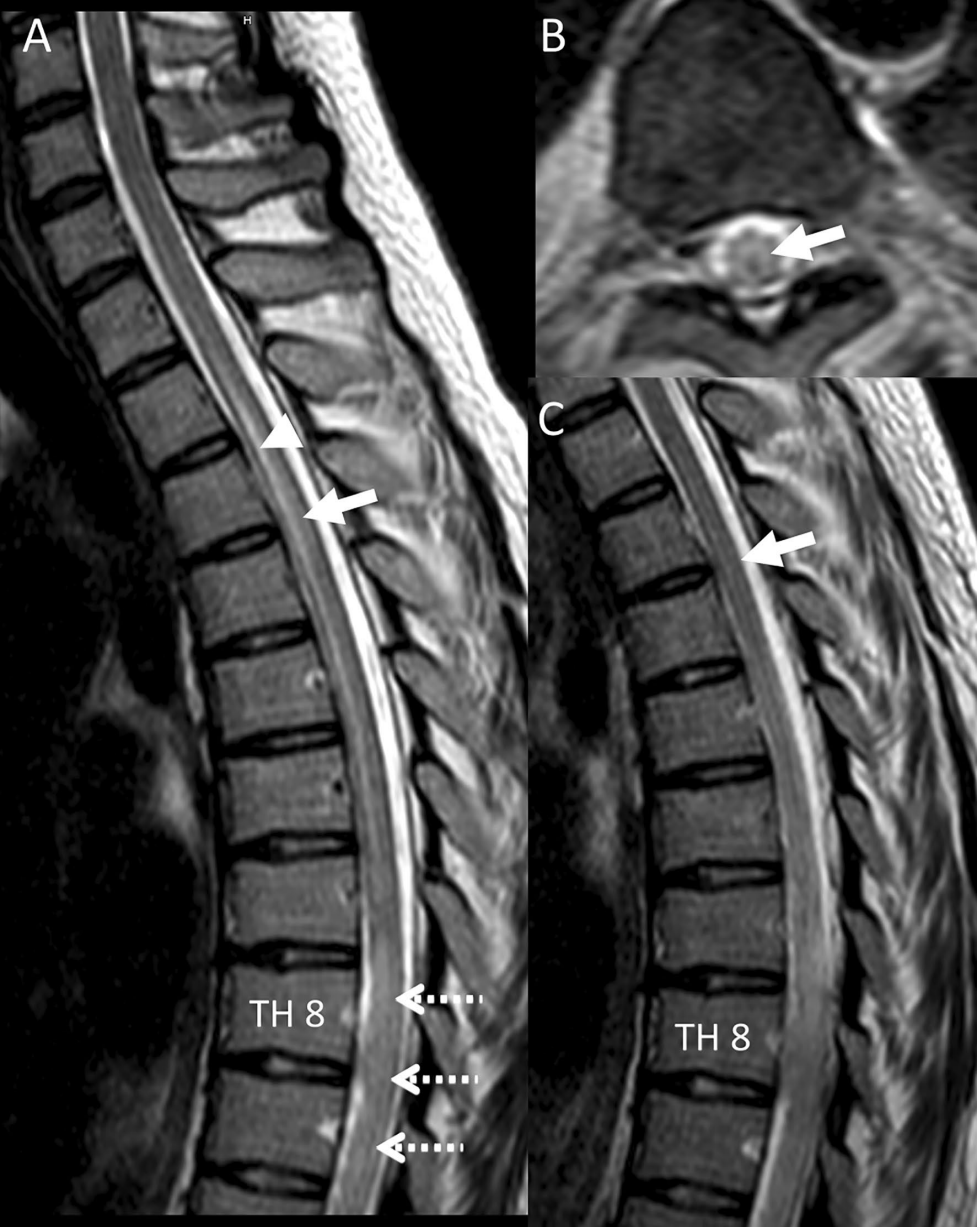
Spinal MRI shows longitudinal thoracic myelitis. Spinal MRI (1.5T, T2w) revealed longitudinal centrally located T2w signal intensities throughout the thoracic myelon (A, lucencies marked by white arrow on sagittal images) indicating an extensive thoracic myelitis with maximal extent at TH7-10 on axial (B, 1 week later) and sagittal (C) T2w images. In the acute stage (A), the central canal (white arrowhead) was slightly enlarged in the thoracic myelon (A) above the maximal swelling at Th7–10 (white dashed arrows) which slightly reversed on follow-up MRI (1 week later; B, C). However, severe longitudinal myelitis persisted (C); note the centrally located inflammation of the thoracic myelon on the axial slice (B)
